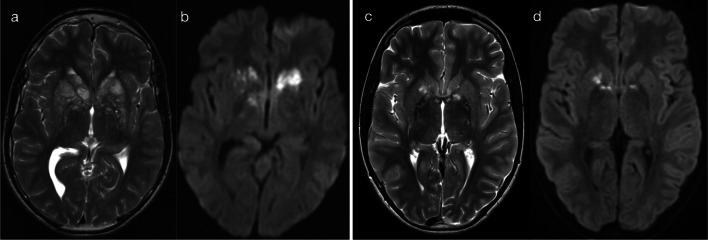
T2 hyperintensity in basal ganglia and/or thalami (Pattern 5). Case 1: 15-year-old child with headache, nausea and vomiting. CSF was positive for cryptococcus. T2-weighted imaging (a) demonstrated bubble-like lesions within the basal ganglia (relating to gelatinous pseudocysts), with a relatively smaller component of restricted diffusion (b). Case 2: 14-year-old child with malaise for 2 weeks. CSF was positive for cryptococcus. T2-weighted imaging (c) demonstrated bubble-like lesions within the basal ganglia and thalami, with a relatively smaller component of restricted diffusion (d)
